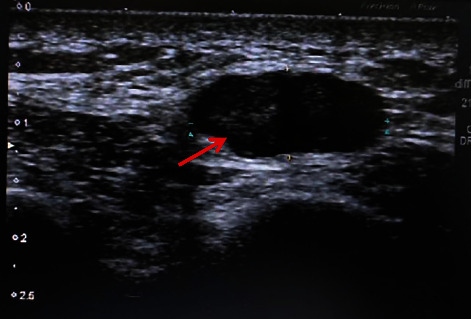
This is the chest wall ultrasound of patient 5. That showed a chest wall recurrence a year after the initial surgery, presented with occupation and irregular margins.
